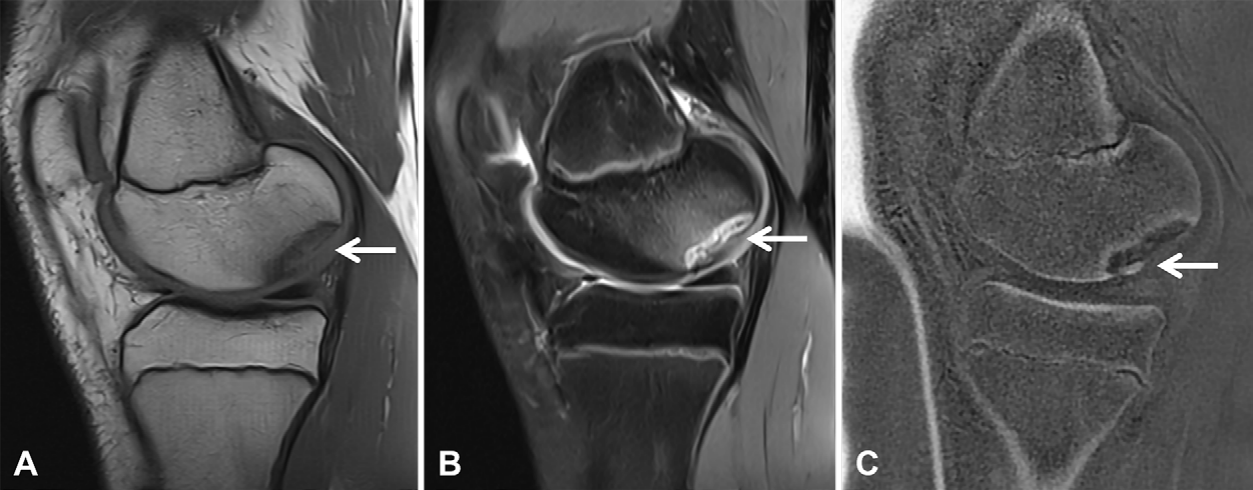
A 15-year-old male soccer player with right knee giving way. Sagittal MRI of the knee demonstrates a large osteochondral lesion (arrows). (A) Sagittal T1-weighted and (B) fat-suppressed proton density images show a focal osteochondral defect in the medial femoral condyle with associated cartilage involvement and subchondral bone marrow edema (arrows). (C) The ZTE image provides improved delineation of the subchondral bone, demonstrating a fragmented cortical fragment (arrow).
